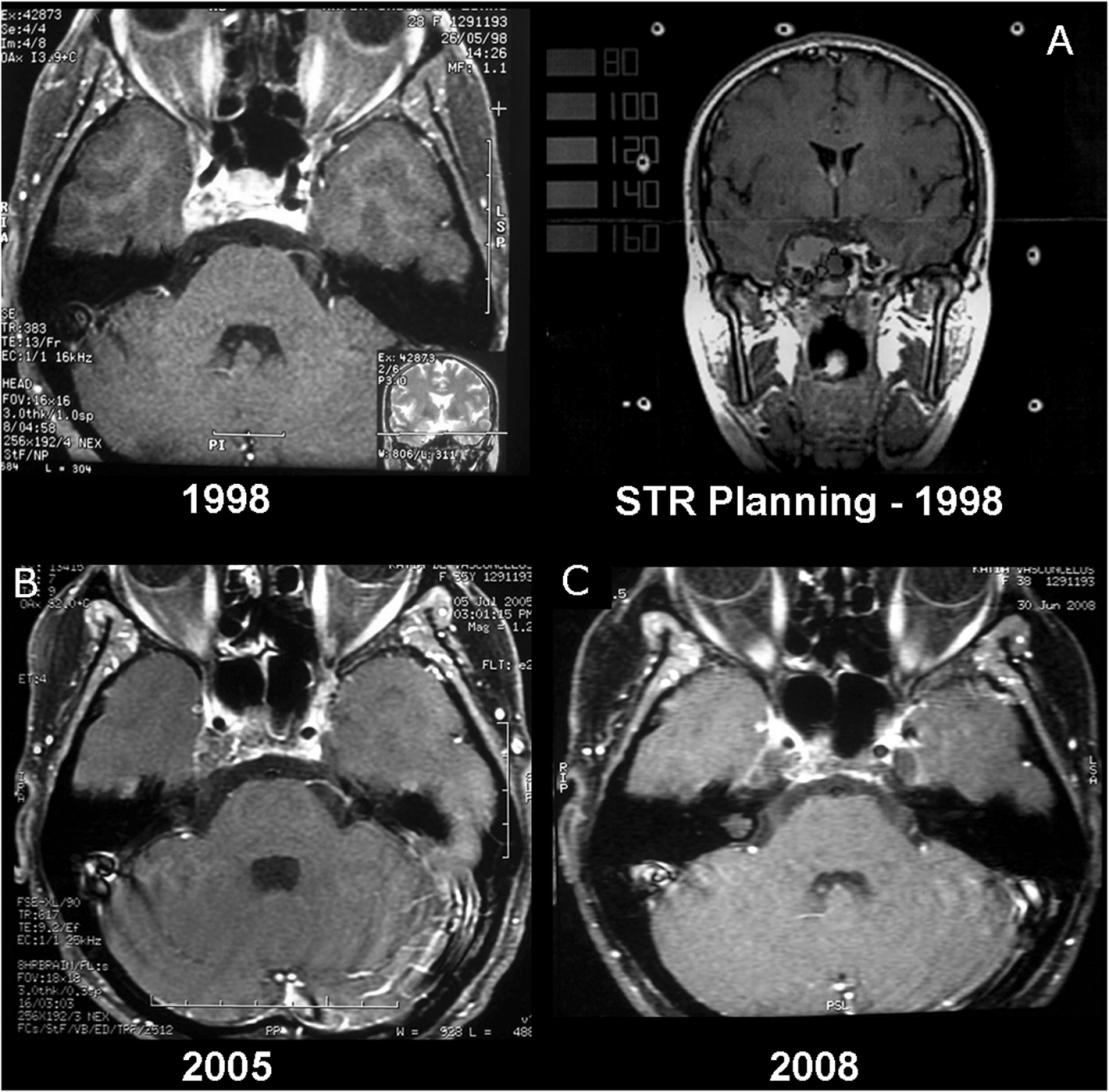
MR images demonstrating radiological evolution in 10 years by STR treatment of patients with CV meningiomas. A: MRI for STR planning (1998); B: MRI after 5 years of the STR; C: MRI after 9 years of the STR.
